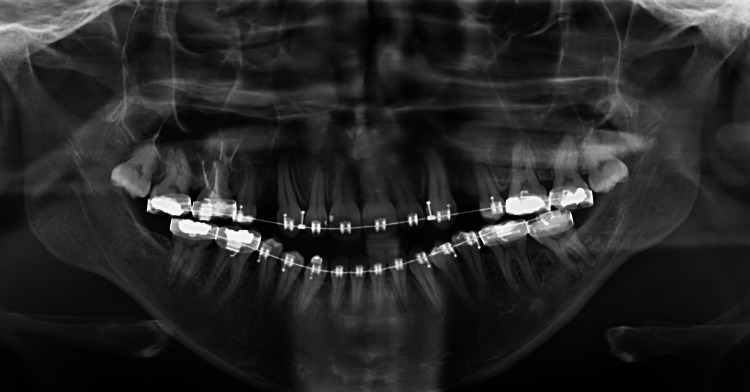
Pre-operative panoramic radiograph. This is a panoramic radiograph of the patient before undergoing the orthognathic surgery which included Le Fort 1 osteotomy, bilateral sagittal split osteotomy, and advancement genioplasty to correct his skeletal and dental disharmony.
